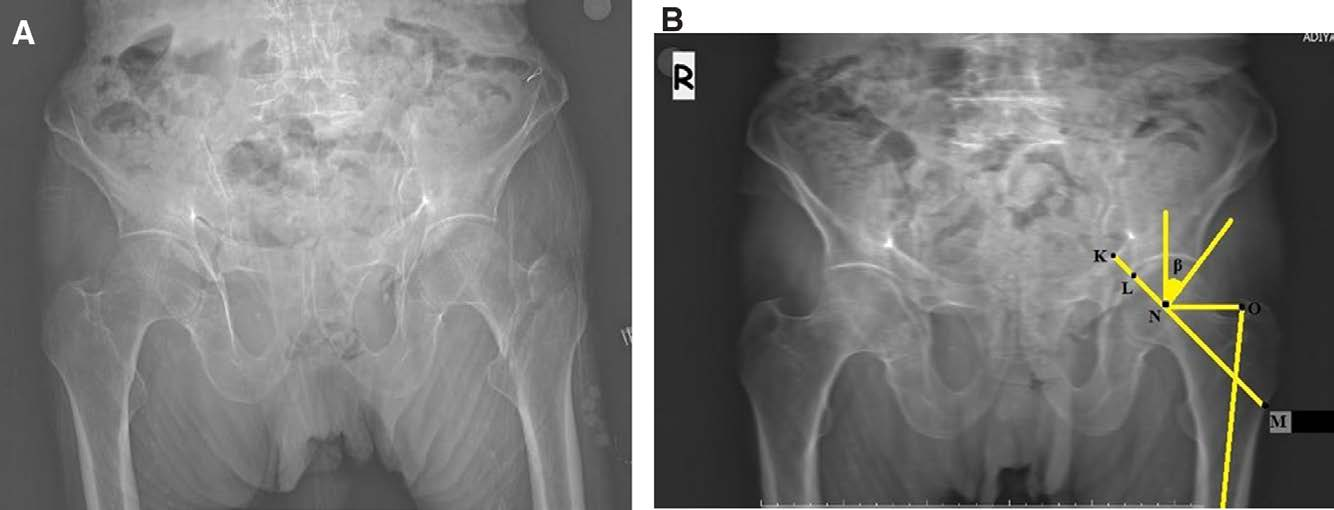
Anteroposterior radiograph of the pelvis shows the central edge angle (β), femoral offset length (line NO), femoral neck axial length (line LM), hip axis length (line KM) (B) and post-traumatic fracture radiograph of the same patient (A).
